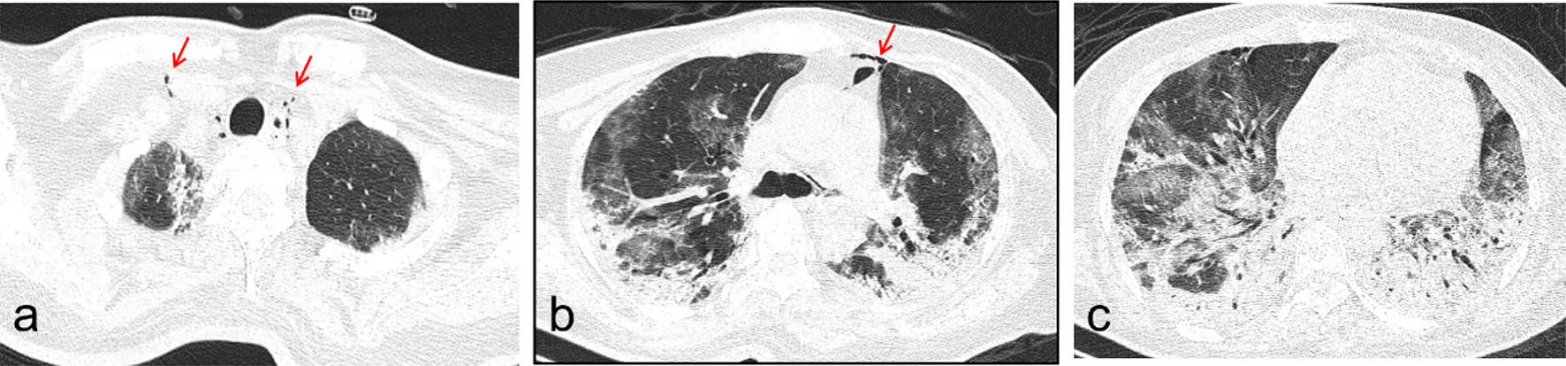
Transverse CT scans in a woman infected with SARS-CoV-2 and suffering type 2 diabetes for 10 years. Scan obtained on 16th day from onset of symptoms shows bilateral, diffuse consolidation, which predominantly involved the lower lobes. There was pneumomediastinum were also seen (a,b arrows). The patient died 7 days after this scan because of both circulatory and respiratory failure.
